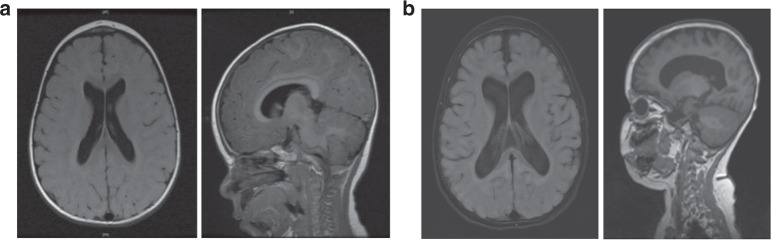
Magnetic resonance imaging (MRI) of patient 1 demonstrating progressive cerebral atrophy.
